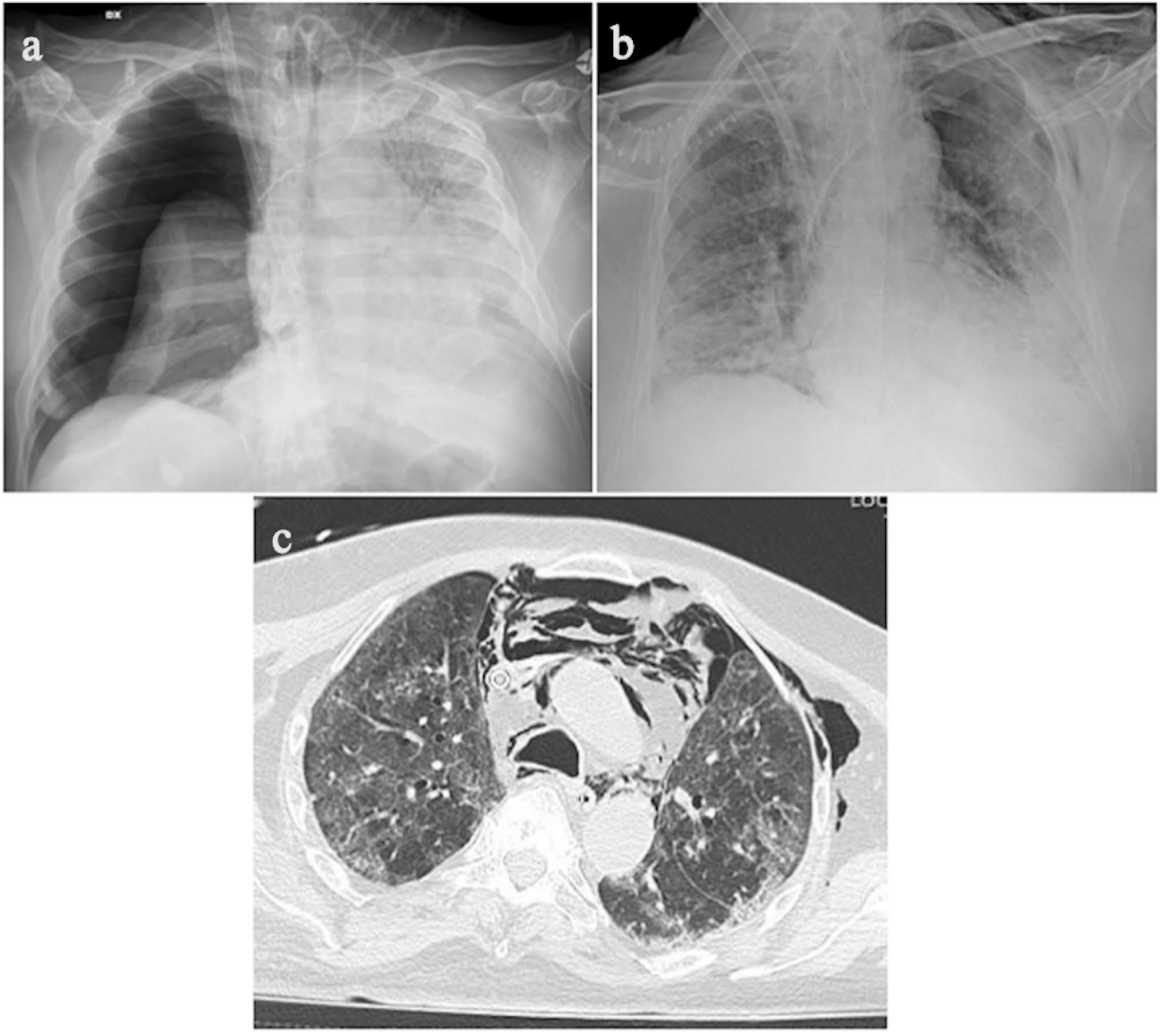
a Chest radiograph showing right pneumothorax. b Chest radiograph and c axial CT image showing pneumomediastinum, left pneumothorax and left subcutaneous emphysema
